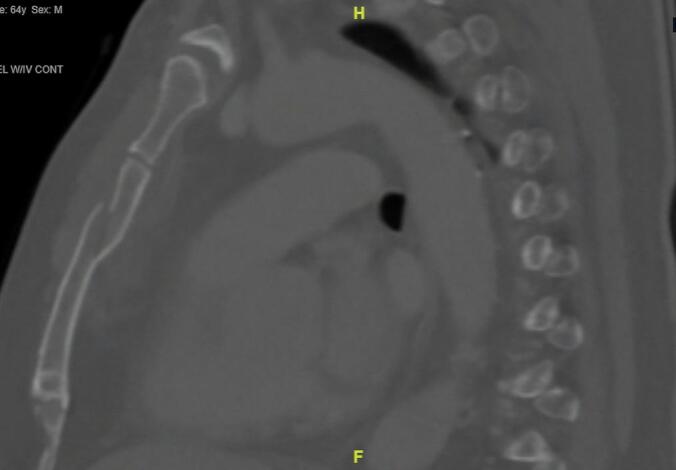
Preoperative CT scan demonstrating displaced, oblique sternal fracture in Patient 2.
